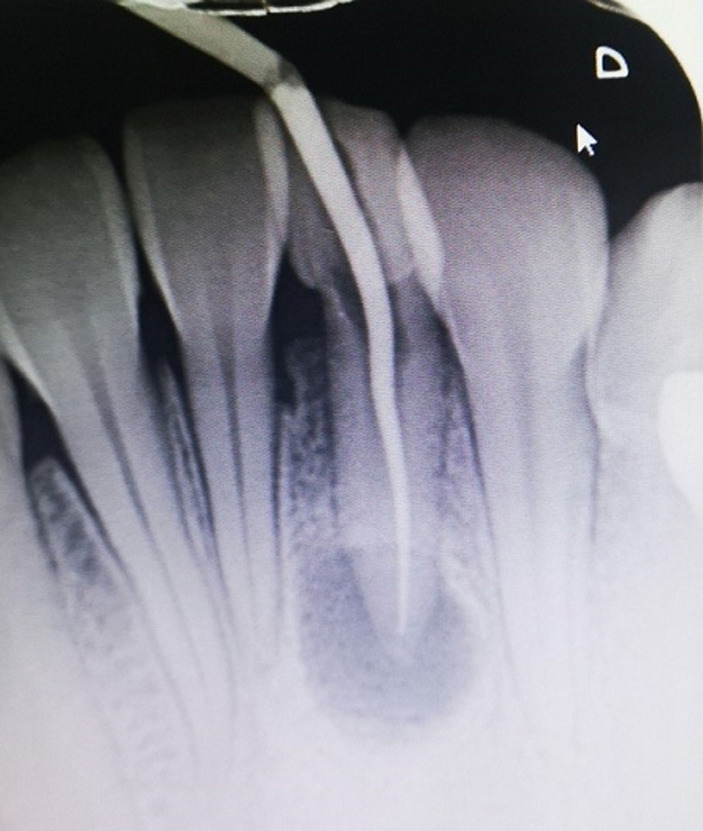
periapical radiograph showing an apical radiolucency, surrounding the apex of 31
